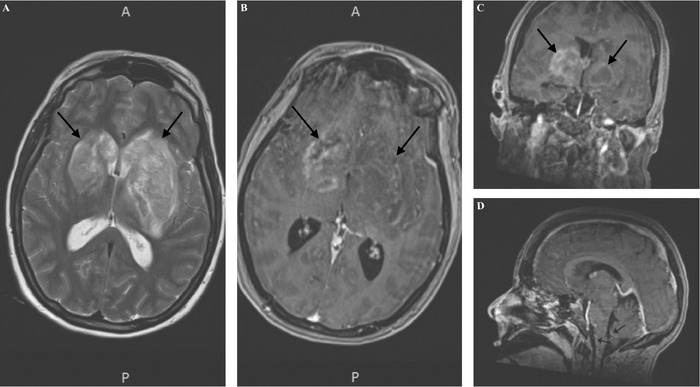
(A-C) Magnetic resonance imaging (MRI) brain with contrast shows peripheral enhancement of the basal ganglia lesion and new progression across the midline involving the right basal ganglia extending to the right cerebral peduncle (arrows). (D) MRI brain shows leptomeningeal enhancement of the superficial surface of the brainstem and proximal cervical spinal cord (arrows).
